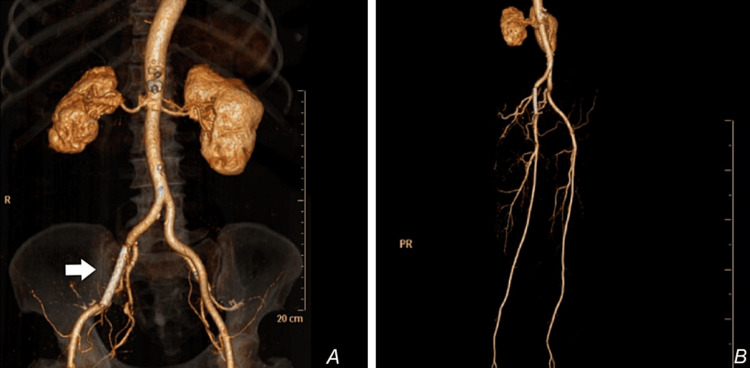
Post-procedure reconstructed CT angiogram of the lower limbs (A) Good stent position (white arrow) and no residual leak. (B) Good distal perfusion and patent stent.
